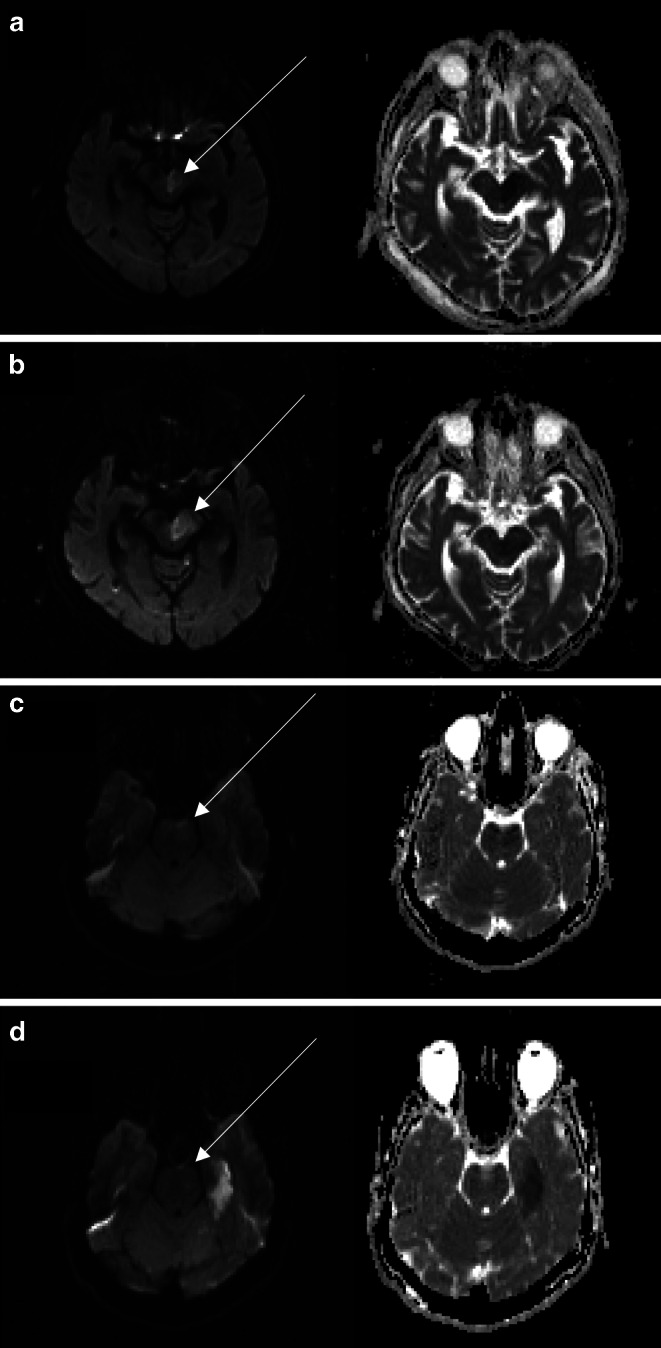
patient with acute ischemic stroke (AIS) due to basilar artery occlusion (BAO) DWI imaging b1000 image and correlating ADC Map before (a) and after (b) successful recanalization of the basilar artery: this patient showed no DWI lesion reversibility, but instead a growing left-sided mesencephalic infarction (arrows) The second patient with AIS due to BAO before (c) and after successful recanalization (d)—this patient showed a reversible left-sided pontin DWI lesion after recanalization and new or growing ischemic cerebellar and left mesotemporal lesions (arrows)
